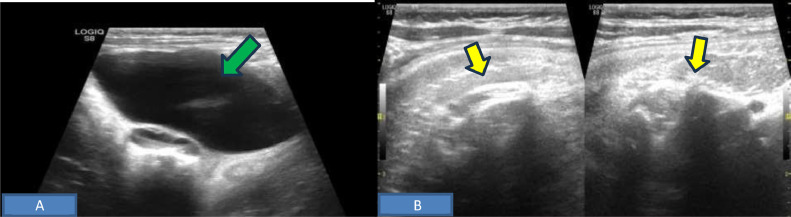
Abdominal ultrasound* demonstrates complex lesion in the left hypochondrium with cystic component (A) and 2 echogenic line casting dense posterior acoustic shadowing and (B) possibly due to fetal spine.
